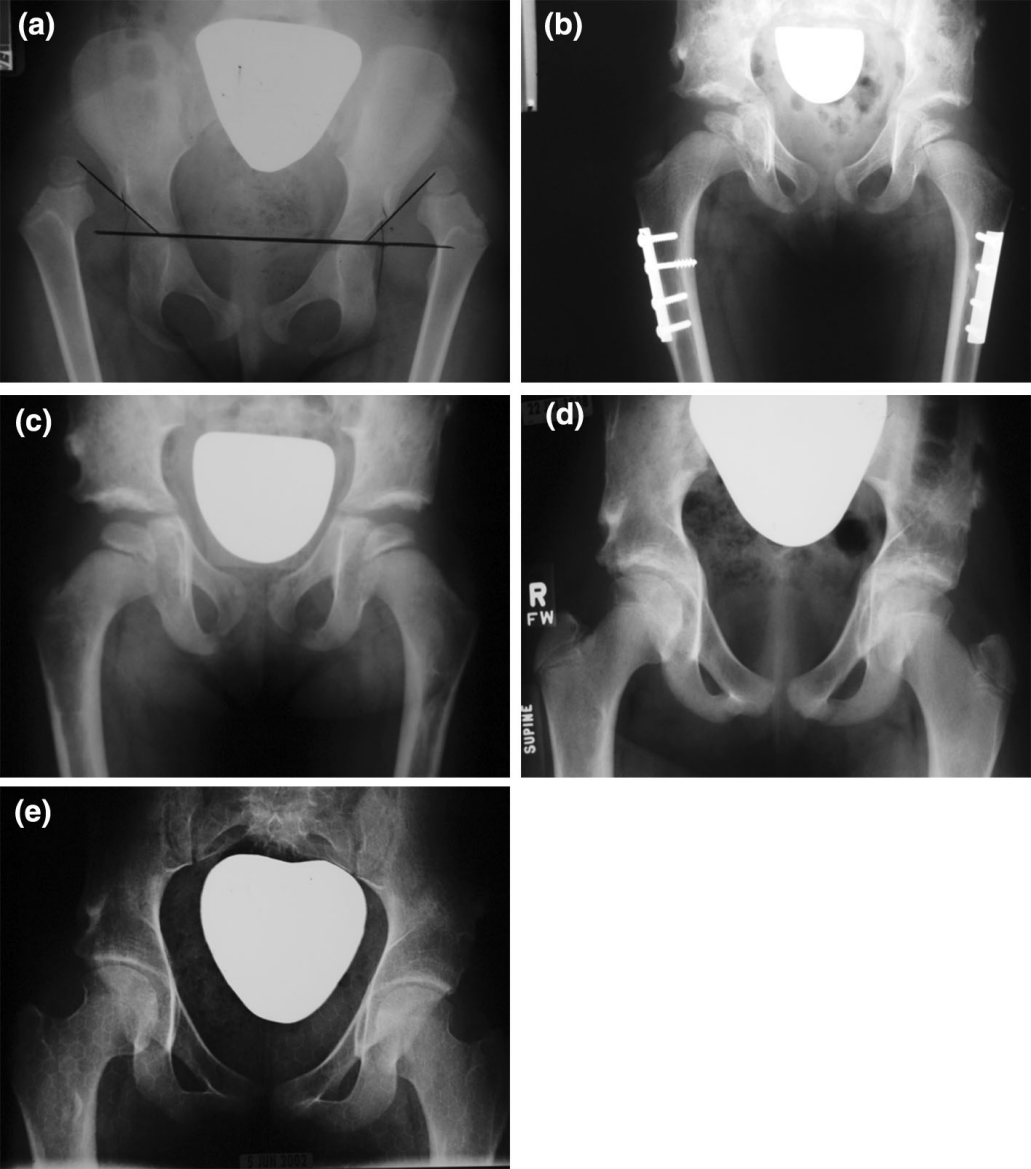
a X-rays of young girl with bilateral dysplasia of the hip (DDH) (Tonnis Grade 4) who underwent surgery at 3 years and 6 months of age. a Preoperative X-ray anterior-posterior (AP) view, b post-operative AP radiograph at 2 years of follow-up after bilateral open reduction (OR), capsulloraphy (Cap), Dega osteotomy, and femoral shortening (FS), c AP radiograph at 6 years of follow-up after plate removal, with excellent head coverage and full range of motion, d post-operative AP radiograph at 12 years of follow-up, e postoperative AP view at 21 years of follow-up with both radiographic and clinical excellent results and no signs of hip arthrosis
